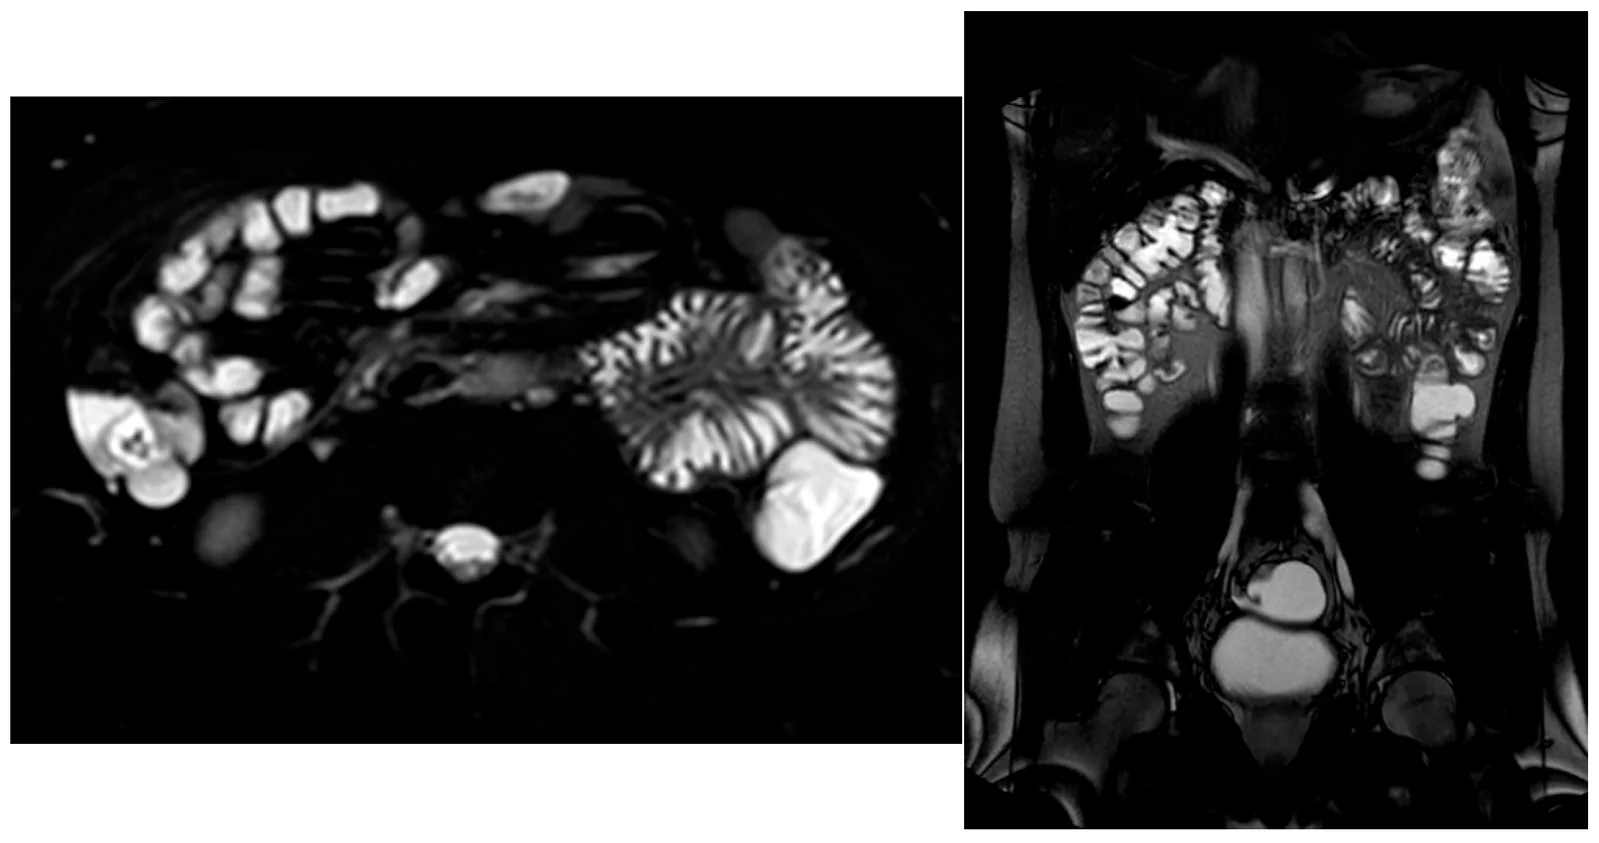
Follow-up magnetic resonance enterography showing complete resolution of the jejunal wall thickening and disappearance of ascites. Images include coronal and axial T2-weighted sequences.
